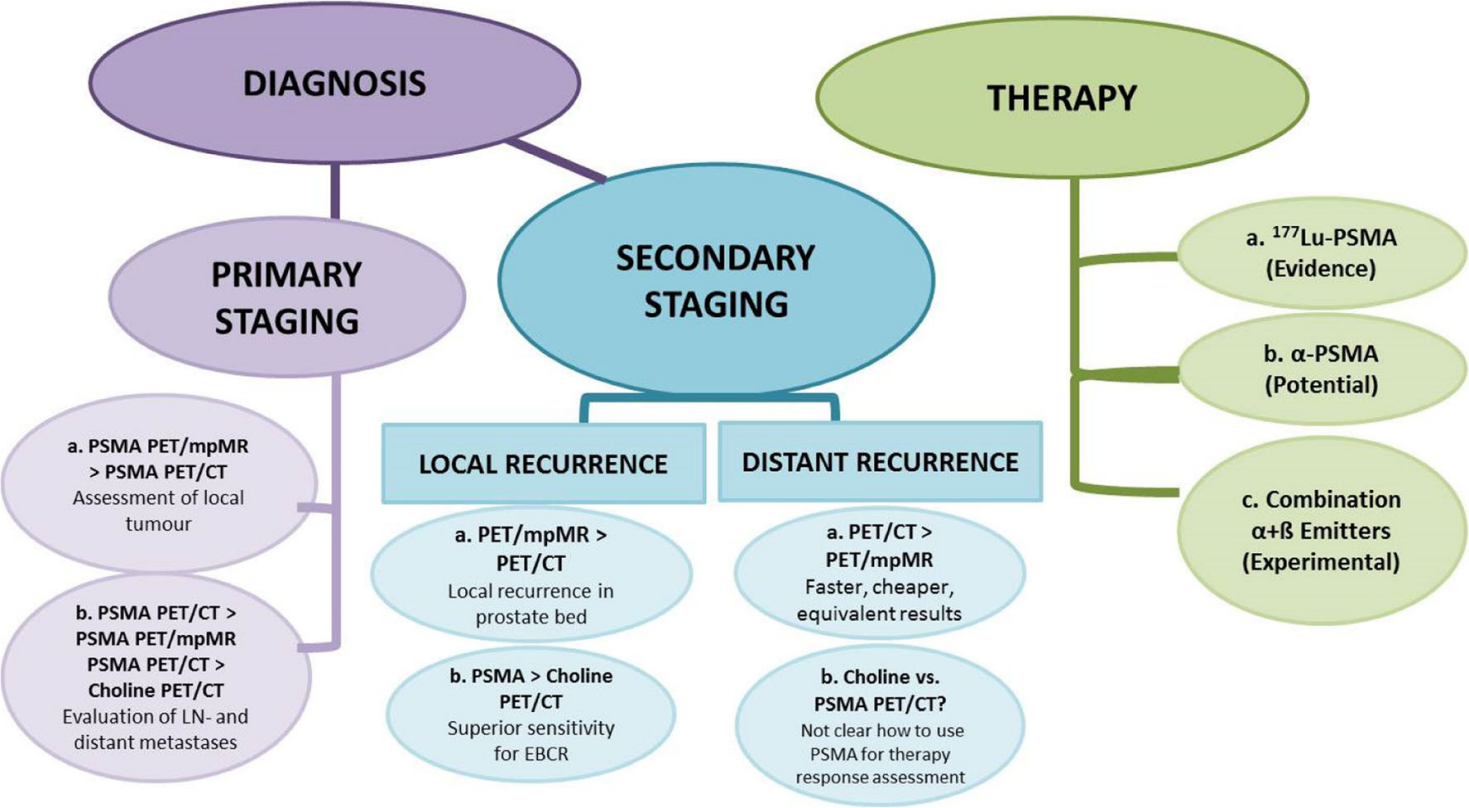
Current status of theranostics in prostate cancer ("Prostanostics"). The current status of PSMA-directed theranostics in PC patients is based on retrospective studies. ^68^Ga-PSMA PET/CT in primary staging is meaningful in patients with high-risk PC for local tumor assessment. The combination with pelvic multi parametric (mp)MR (or PET/mpMR) reaches the highest impact on patient management. In secondary staging for local recurrence, ^68^Ga-PSMA PET/mpMR is superior to PET/CT, whereas for distant recurrence, PET/CT has equivalent results and is faster and cheaper compared to PET/mpMR. ^68^Ga-PSMA PET/CT is superior to ^18^F / ^11^choline PET/CT in primary staging as well as in secondary staging. Significant clinical results have so far been achieved with the subsequent use of radiolabeled PSMA ligands in the treatment of CRPC, especially with ^177^Lu-PSMA ligands. Potential results have been demonstrated for α-emitting ligands and the combination treatment of β- and α-emitters is discussed
